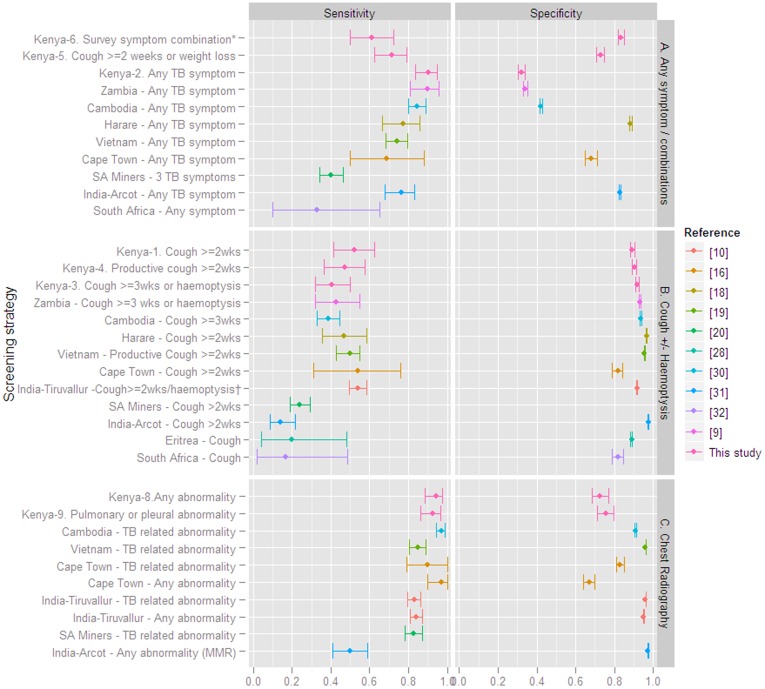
Sensitivity and specificity of symptom and chest radiography screening in population based TB screening (10 prevalence surveys [9], [10], [13], [16], [18], [19], [28], [30], [31], [32] including our report and 1 pre-mass IPT screening [20]), reported since the year 2000. Panel A summarizes screening for ‘any TB symptom’ or combinations of 3 or more symptoms. Panel B summarizes screening for cough of ≥2 or ≥3 weeks, and panel C summarizes chest radiography screening. *Kenya-1, Kenya-2 etc. refers to the strategy numbers described in Table 1 and Table 4. †includes also fever >1 month or chest pain. MMR = Mass Miniature Radiography.
